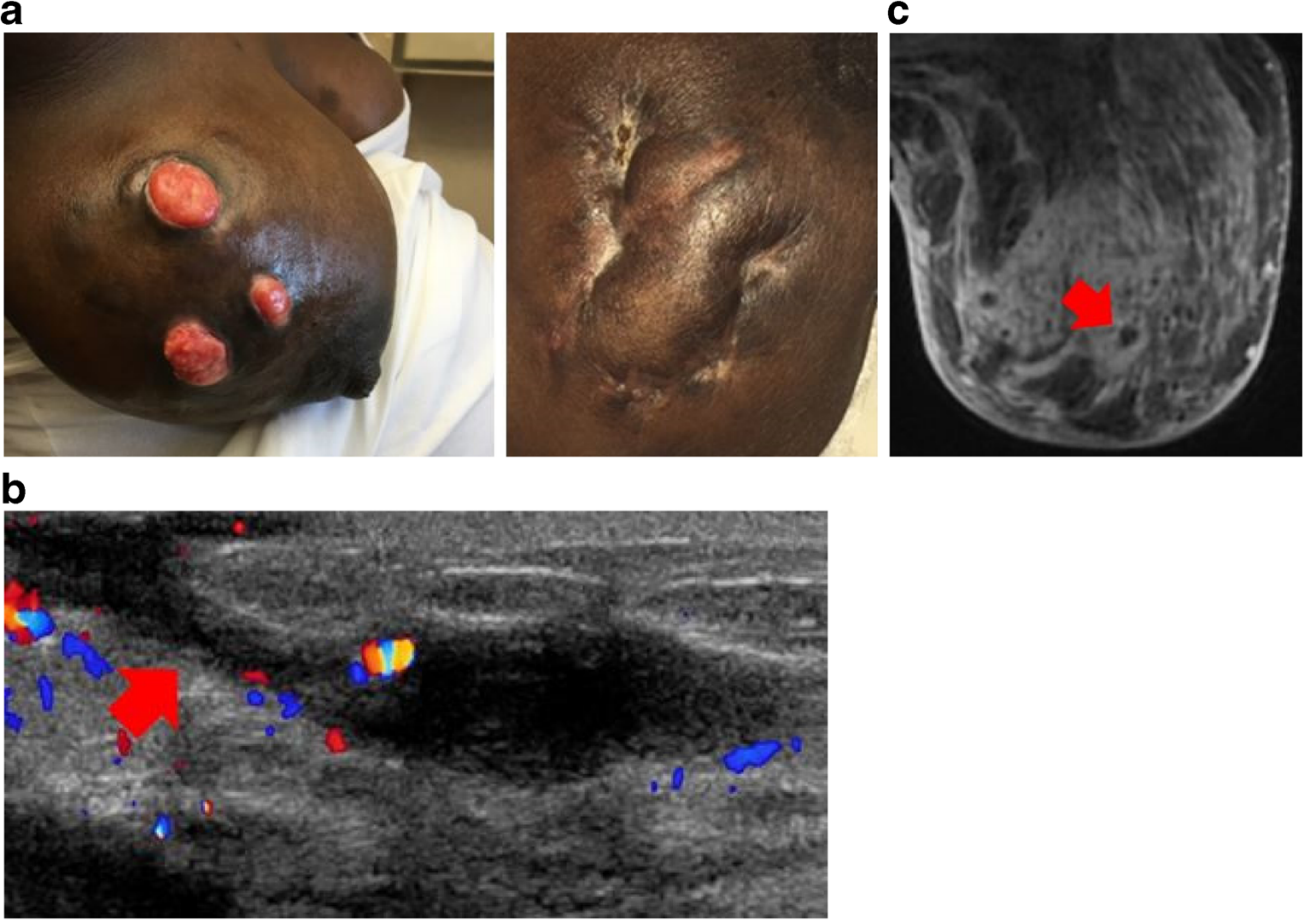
Idiopathic granulomatous mastitis. A 30-year-old woman in the second trimester of pregnancy had a tender lump in the right breast. She had first noticed a lump in the upper outer right breast approximately 2 months previously, and it had increased in size. On physical examination, there was a 10 × 10-cm firm mass in the upper outer quadrant. Biopsies showed acute and chronic granulomatous inflammation with micro-abscesses. All cultures were negative. a Photographs of open wounds that developed after biopsy (*left*) but healed with antibiotics but no steroids (*right*). b Ultrasound showed a large hypoechoic irregular mass with draining sinus tract (*red arrow*). c Axial post-contrast MRI showed diffuse parenchymal enhancement with micro-abscesses (*red arrow*)
